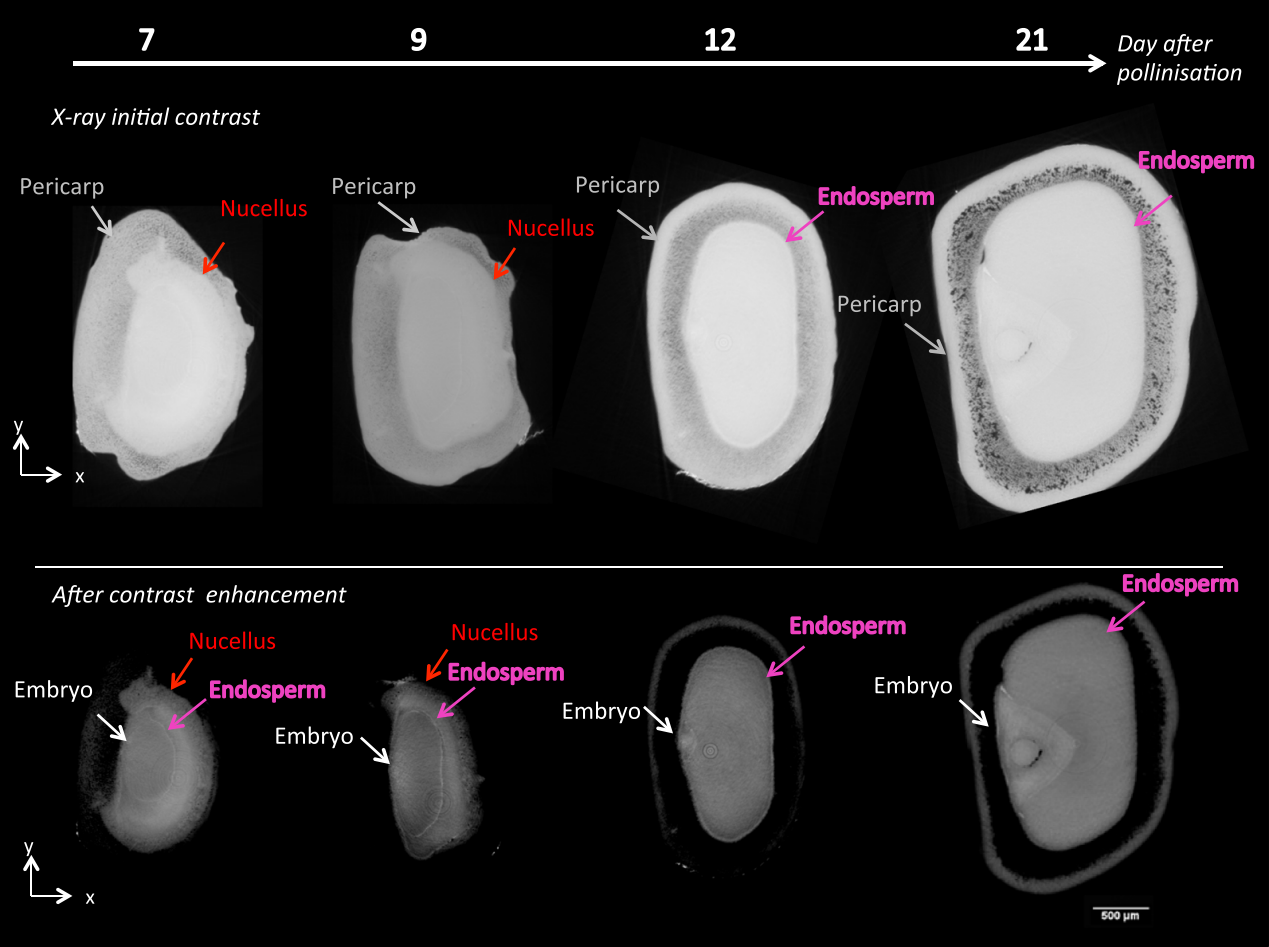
X-ray in-line phase tomography of maize seeds at 4 dates after pollination. *Top line* initial contrast enhancement on the whole *gray* level dynamic. *Bottom line* alternative contrast after dynamic reduction
